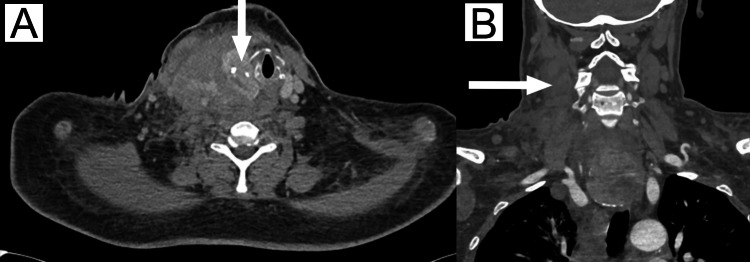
Radiological imaging (A) Axial contrast-enhanced CT image of the neck demonstrating a large right-sided cervical hematoma (arrow) causing marked leftward displacement of the trachea. (B) Coronal reformatted CT image showing the full craniocaudal extent of the hematoma (arrow) extending into the superior mediastinum.
